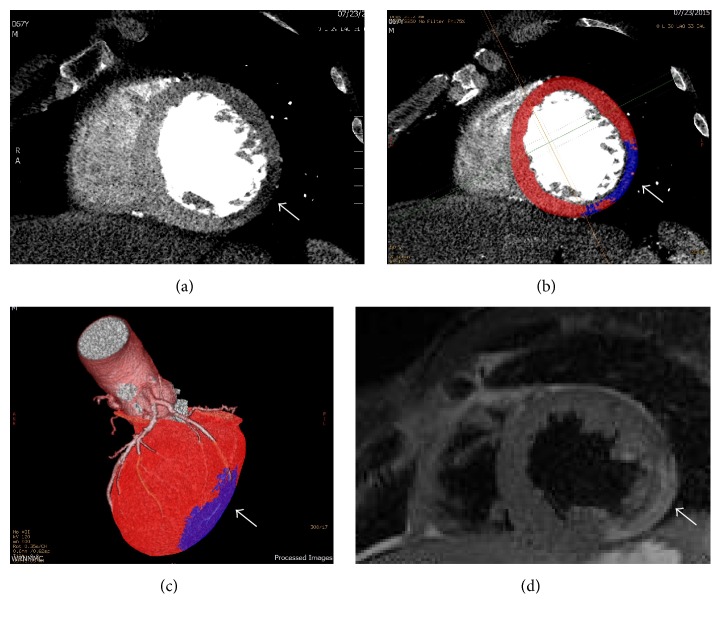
Short-axis first-pass CT perfusion images demonstrating an area of low attenuation of the mid inferolateral wall (a, b, c) corresponding to infarct on cardiac MRI delayed enhancement (d) images.
